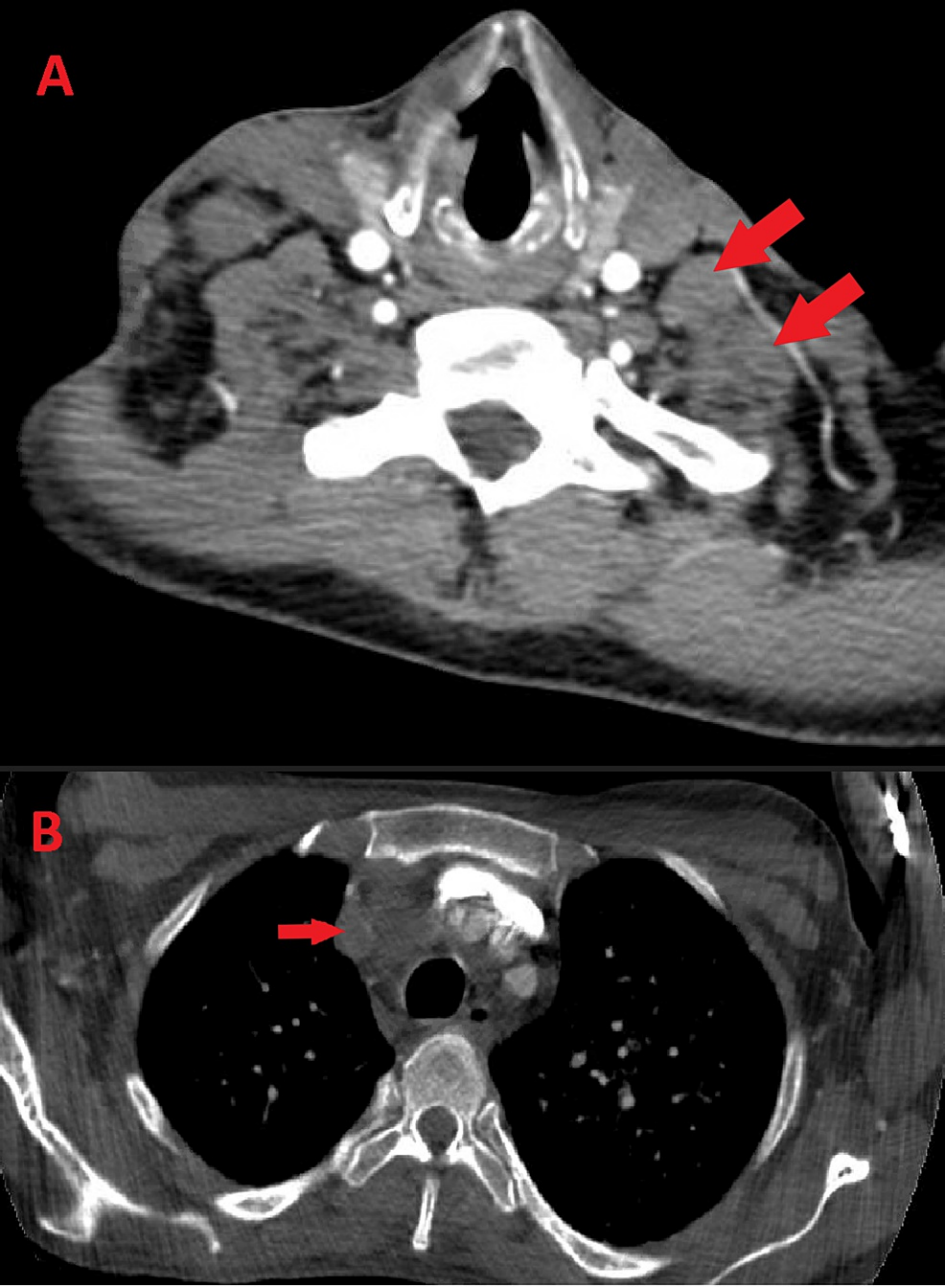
CT images of the chest showing (A) supraclavicular lymphadenopathy and (B) mediastinal lymphadenopathy
